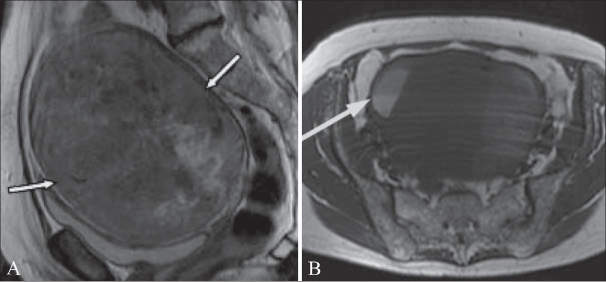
A 70-year-old woman with postmenopausal bleeding and a pelvic mass. Sagittal T2W MRI image (A) shows a 13.5-cm heterogeneous pelvic mass (arrows). Axial T1 MRI image (B) shows areas of high signal within the mass, including an area with a fluid-fluid level (arrow) that is in keeping with hemorrhage. The patient underwent a hysterectomy and this was confirmed to be a leiomyosarcoma
